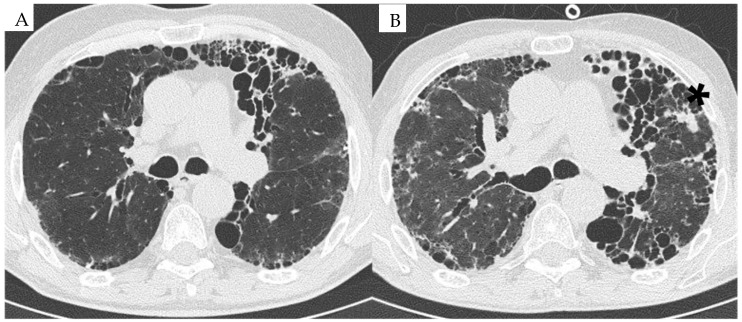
HRCT image of a patient with RA-ILD at baseline in 2015 (A) and follow-up in 2023 (B). (A) UIP pattern associated with RA associated with reticulations and mild ground-glass opacities. (B) Progression of the UIP pattern with the occurrence of a neoplastic lung nodule in the left upper lobe (*).
